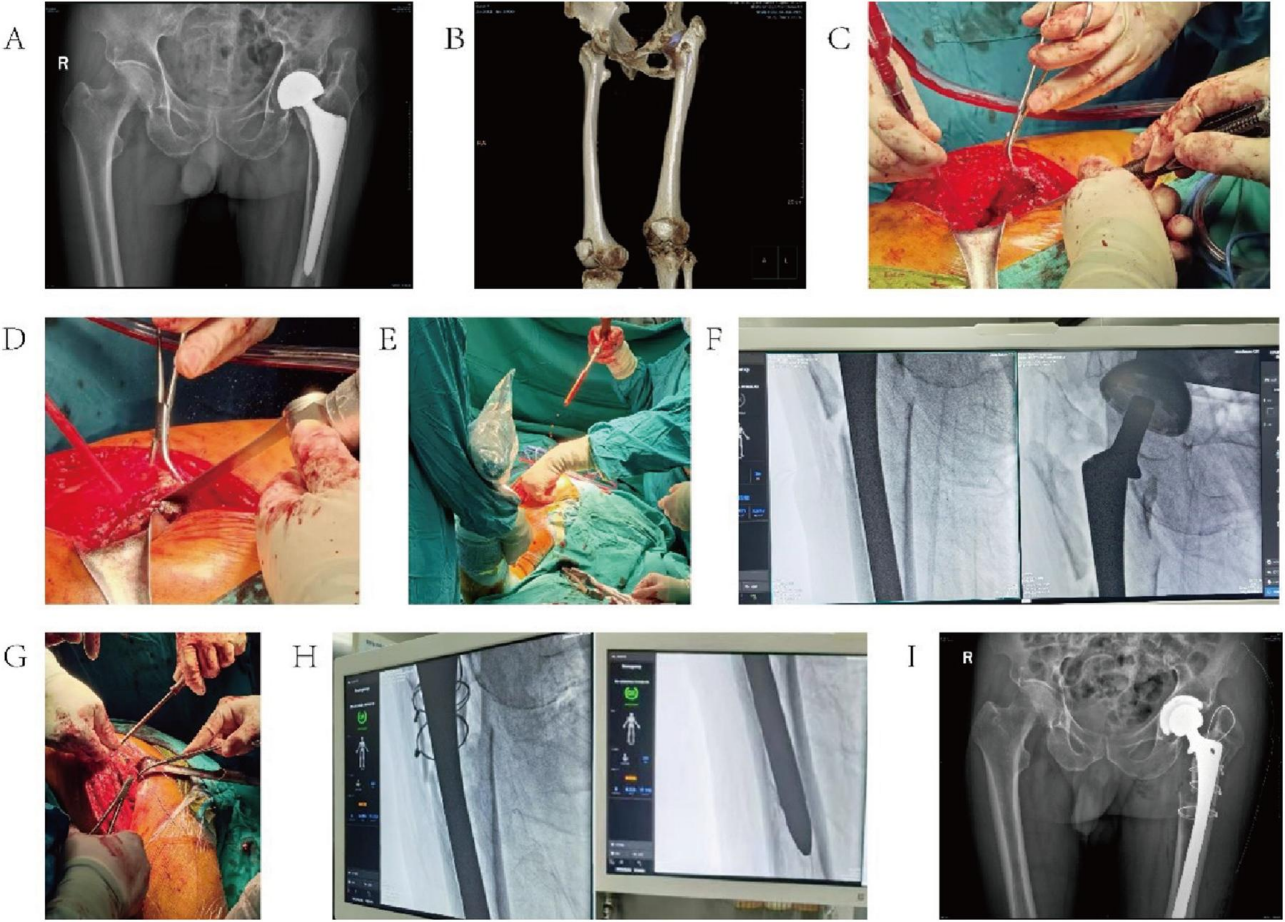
Typical case 2. (A) Preoperative hip X-ray; (B) preoperative CT; (C) scraper to clean the periprosthetic conjunctival tissue around the femur; (D) pendulum saw to remove the excess bone near the greater trochanter; (E) reamer to expand the medulla oblongata and open the sclerotic band of the distal femur; (F) intraoperative finding of proximal femur fracture; (G) bone grafting of the proximal femur and fixation with sternum wire ties; (H) intraoperative fluoroscopy; and (I) postoperative hip X-rays.
